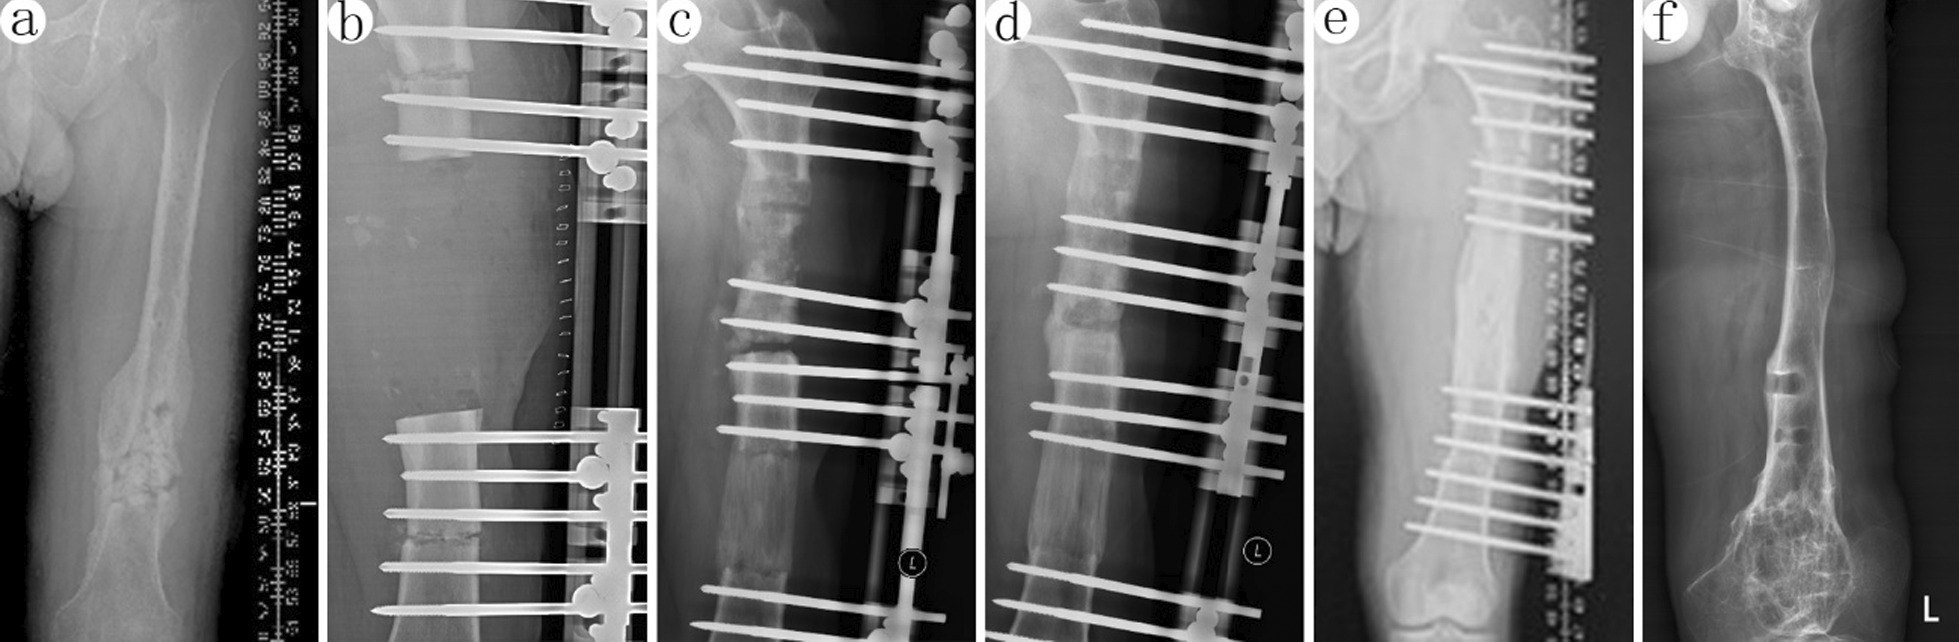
39-year-old male patient with posttraumatic osteomyelitis of the left femur was treated with trifocal bone transport from both side to docking site using Orthofix external fixator. a X-ray graph before treatment. Lesions on the sides of the middle and lower part of the femur. b After debridement, the bone defect reached almost 14 cm. The Orthofix external fixator was used to perform the distal and proximal biplane osteotomy. c The X-ray graphs at 40 days after surgery. The force line was available. d At 80 days, the butt joint healed. Regeneration zone growing well. e Bone healing of the osteotomy line was evident at 8 months. f X-ray film after removing the external frame at last follow-up
